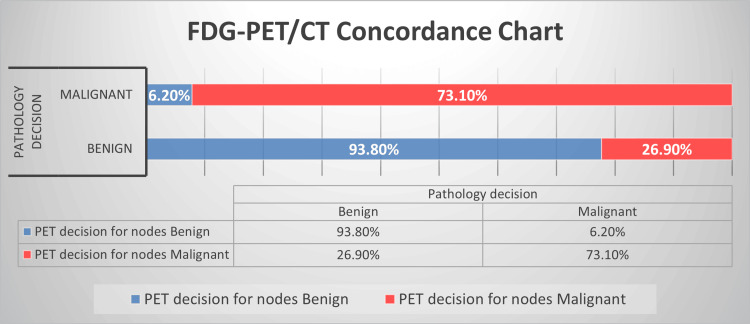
Depiction of FDG-PET/CT concordance with pathology result for subcentimeter nodal involvement in lung carcinoma patients FDG-PET/CT: 18F-fluorodeoxyglucose positron emission tomography/computed tomography
